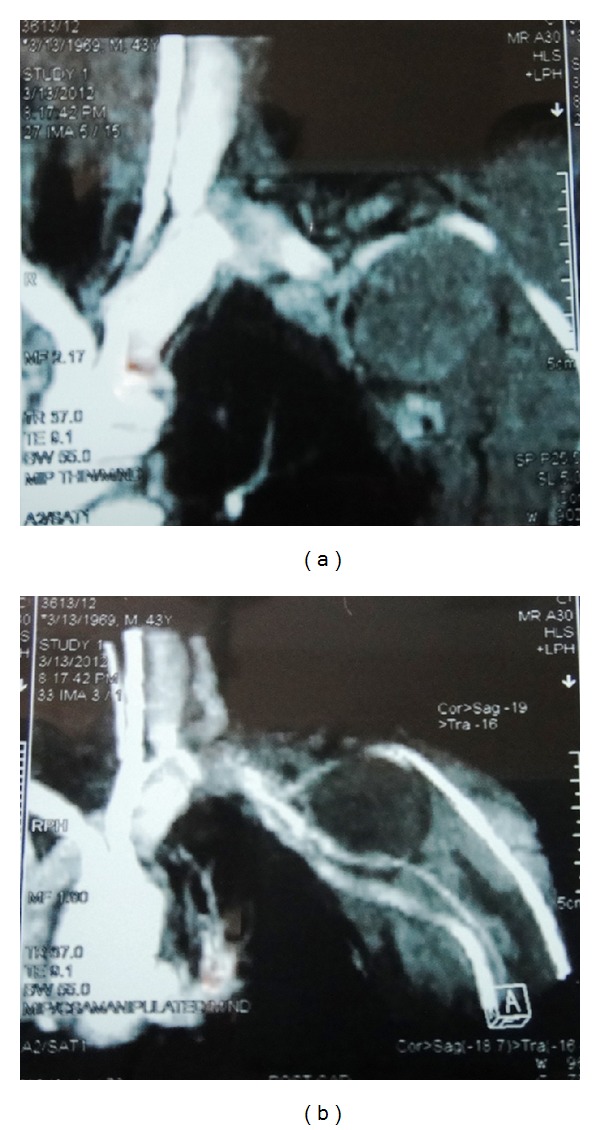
Coronal MRI T1W with gadolinium (a) and (b) images showing a well-defined brachial plexus desmoid tumour that was isointense to muscle on T1W sequences and mixed signal intensity (both low to high signal intensity) on T2W sequences and was straddling the brachial plexus.
